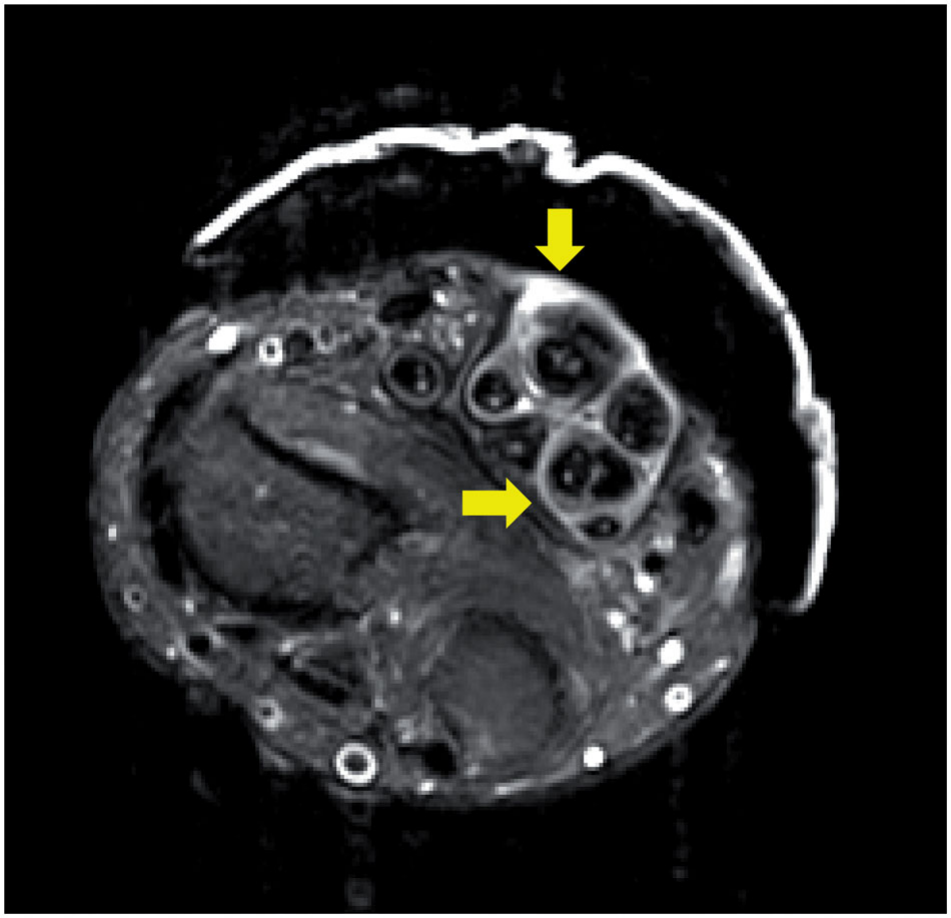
Contrast-enhanced MRI showing hypertrophy of the synovial sheath on the flexor tendon (*arrows*) with T2 fat suppression.
